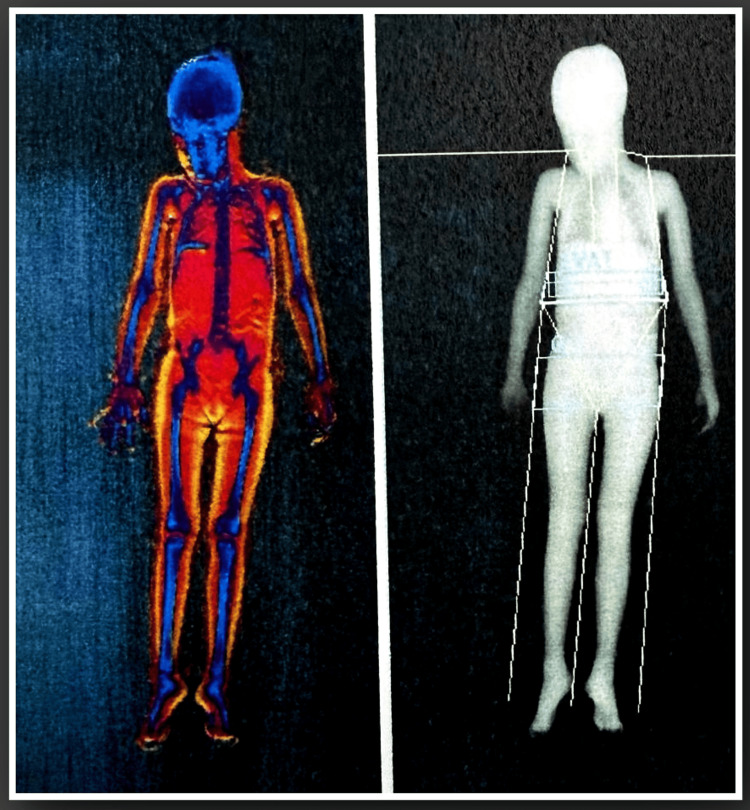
Whole-body technetium-99m bone scintigraphy The scan demonstrates increased radiotracer uptake in multiple long bones, indicating increased bone turnover and supporting skeletal involvement in the clinical context of McCune-Albright syndrome.
